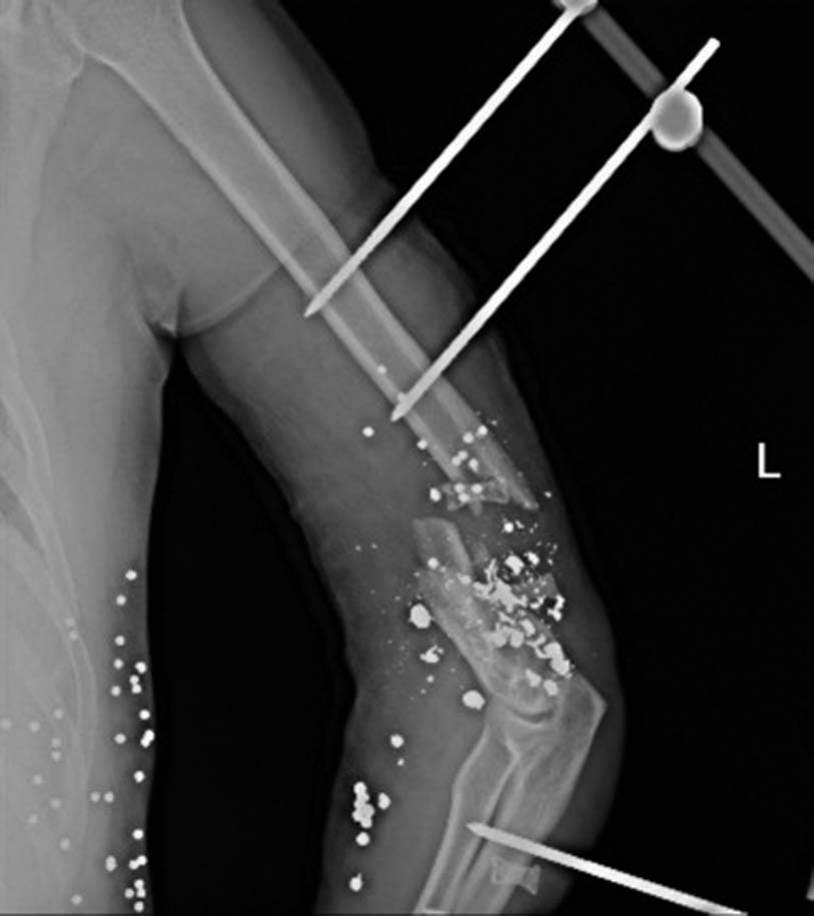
The initial X-ray at admission time, 13A3 fracture fixed with an external fixator in another hospital.
